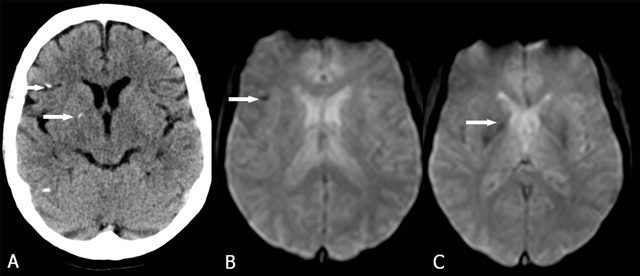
Diffuse axional injury. CT (A) and GRE-T2*(B, C). Multiple small foci of high density (A) corresponding with (arrows) small foci of low signal intensity (B, C) at the borders between the cortical grey and white matter.
